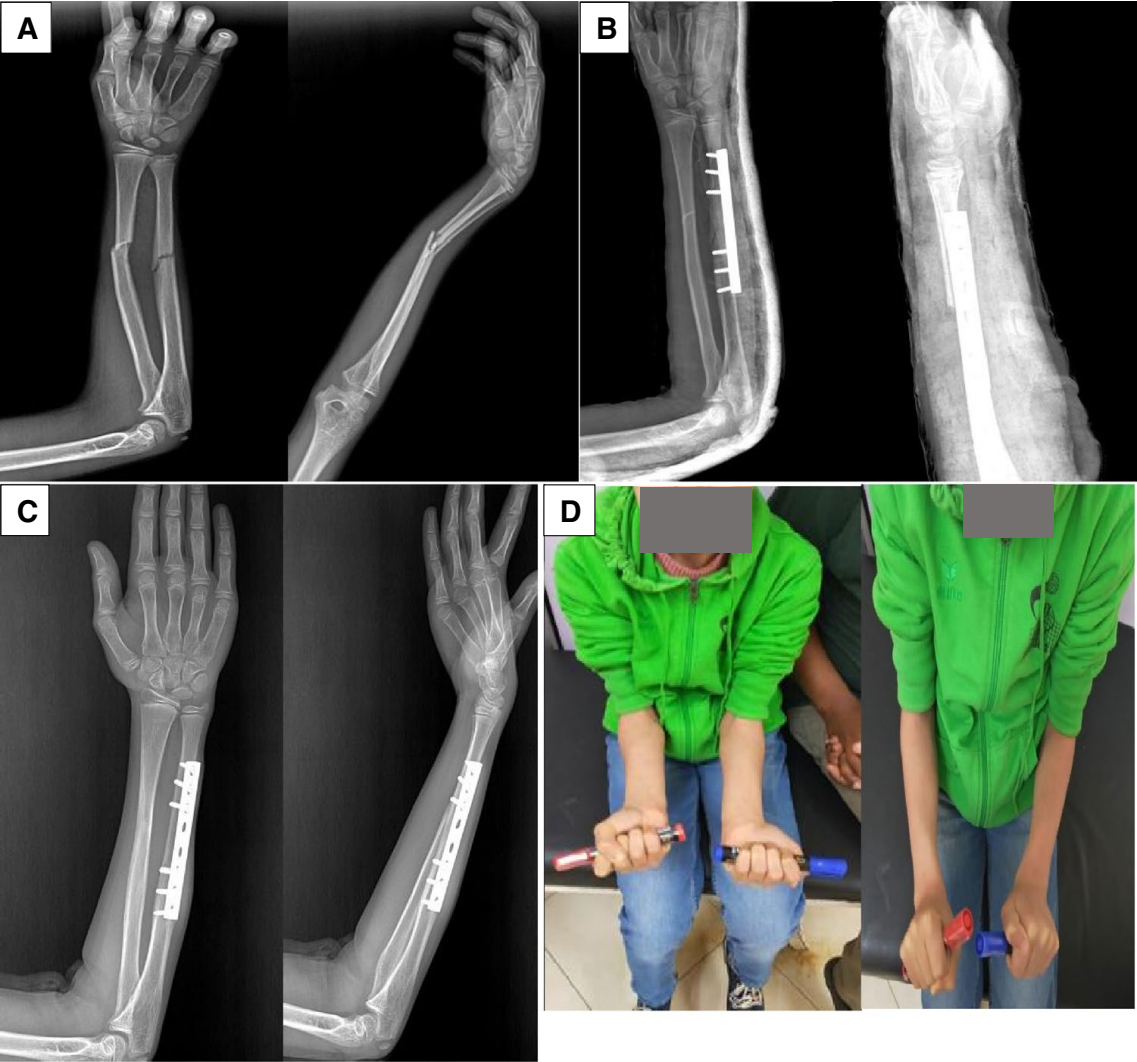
Ten-year-old male child who sustained right both-bone unstable forearm injury following fall down accident. A—Pre-operative radiographs showing mid-shaft forearm fracture with Apex volar angulation. B—Post –operative x-rays (AP & lateral) showing single-bone ulna fixation with well reduced radius. C—X-rays (AP & lateral) at 6-week post-op which confirm radiological union. D—Clinical photograph showing comparable forearm range of motion
